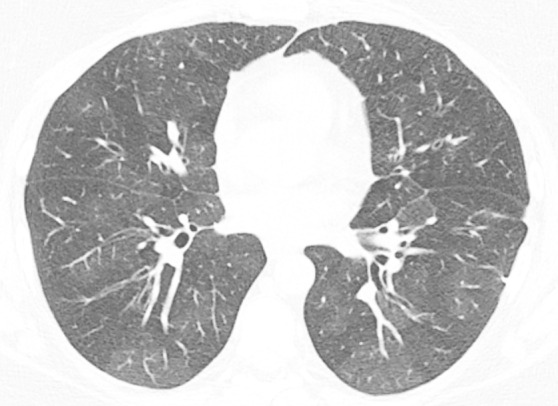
Mosaic attenuation and air trapping. (a) Axial inspiratory CT scan demonstrates mosaic attenuation due to ground-glass opacity in a case of respiratory bronchiolitis-associated interstitial lung disease. (b) Axial inspiratory CT scan demonstrates mosaic attenuation due to small airways disease in a case of constrictive bronchiolitis. (c) Axial expiratory CT imaging (same patient as b) demonstrates accentuation of the areas of hyperlucency from the adjacent lung, confirming small airways disease as the cause of mosaic attenuation.
